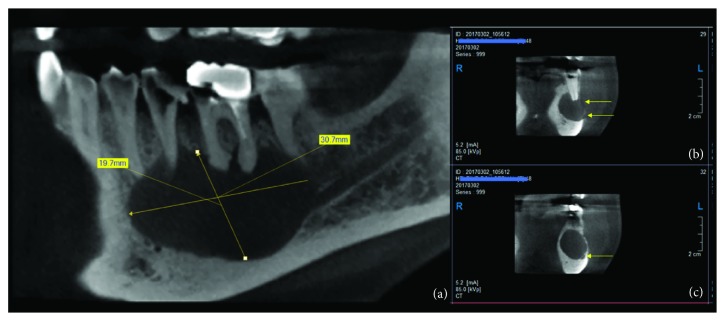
CBCT reconstruction of the left mandible. (a) The sagittal reconstruction with the approximative measurement of the bone destruction (30.7 mm × 19.7 mm). (b) Para-axial cut showing the discontinuity of the buccal cortical bone. (c) Para-axial cut showing the position of the mandibular canal.
